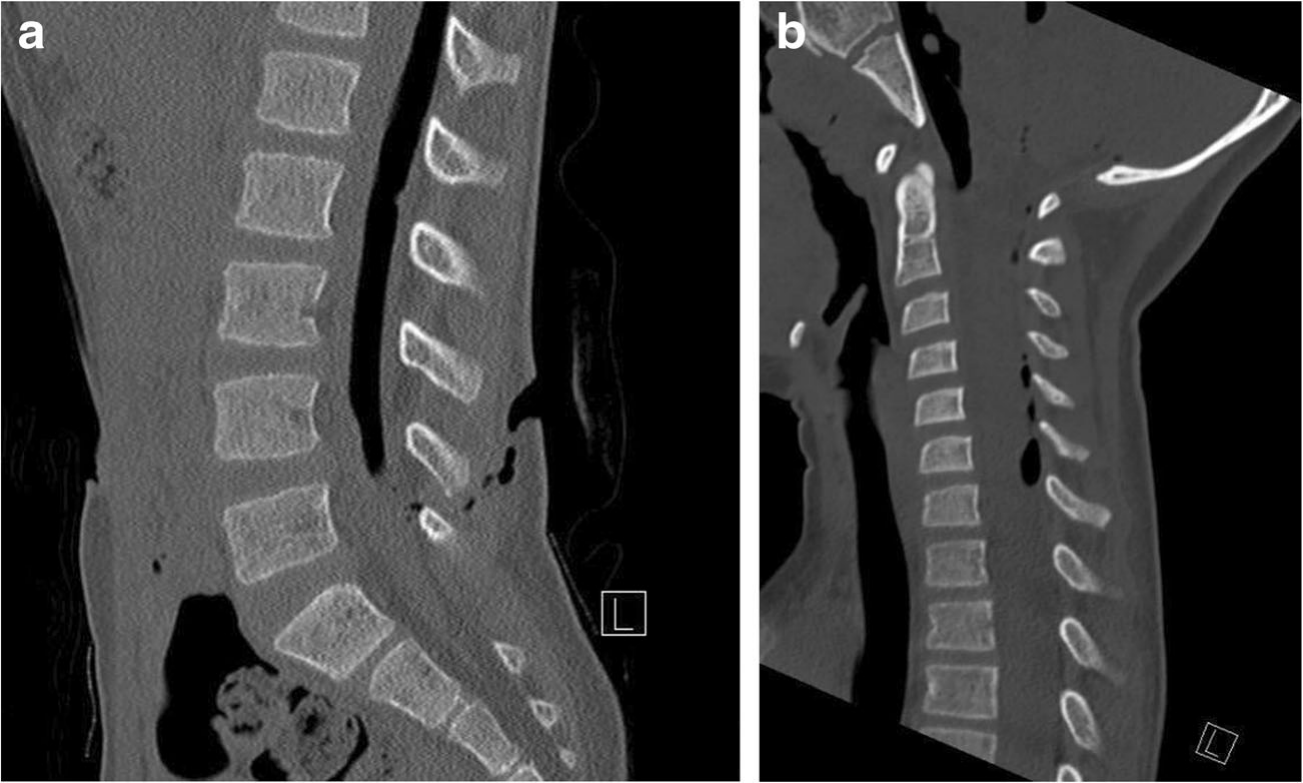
a Lumbar CT, sagittal section. b Cervical CT, sagittal section
